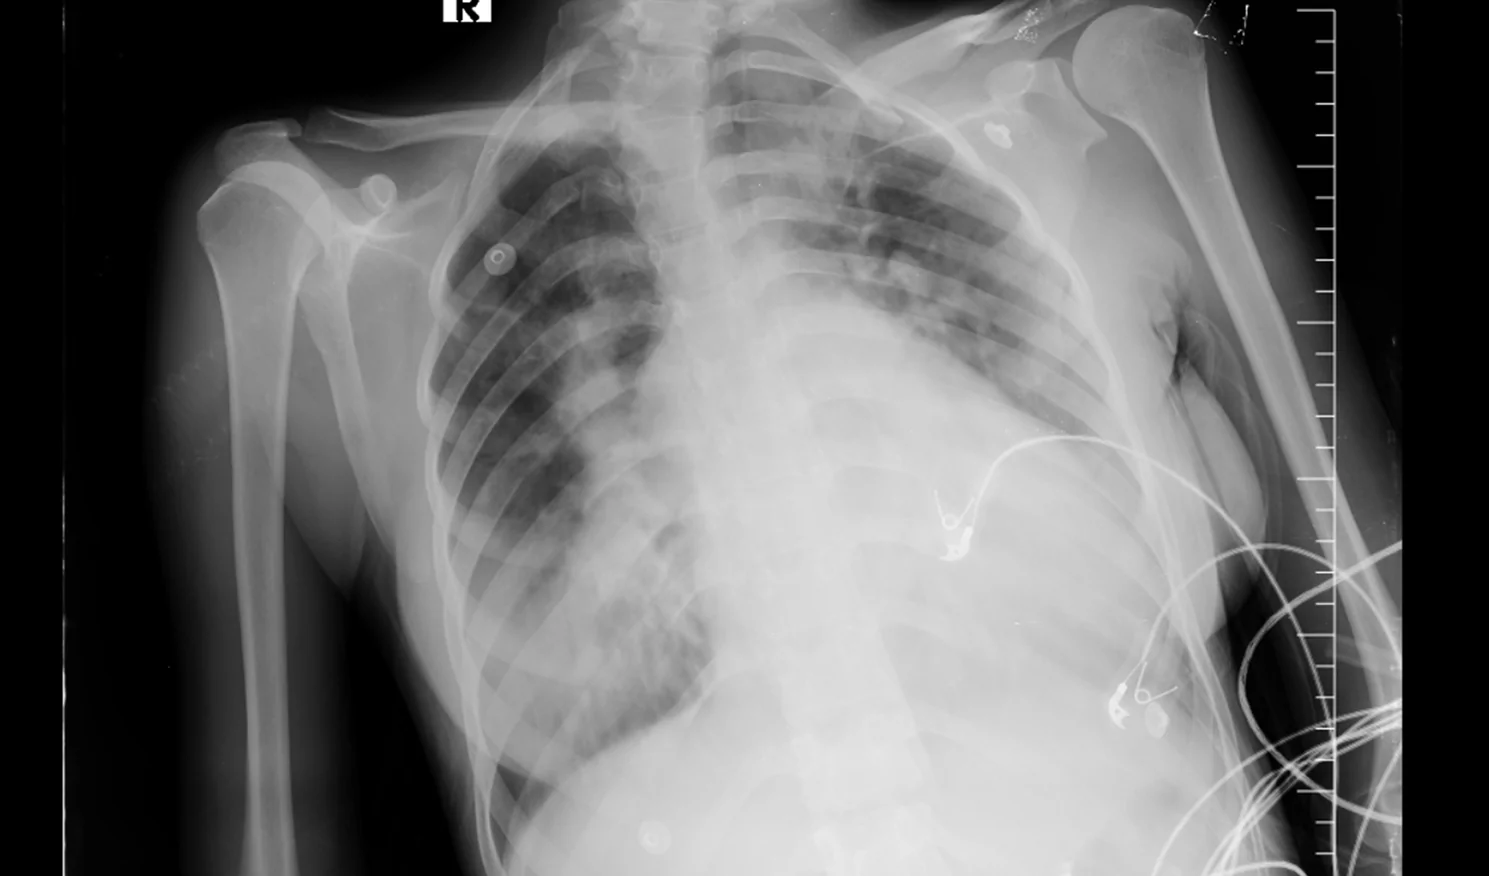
Chest radiograph showing cardiomegaly and bilateral pulmonary vascular/interstitial congestion
